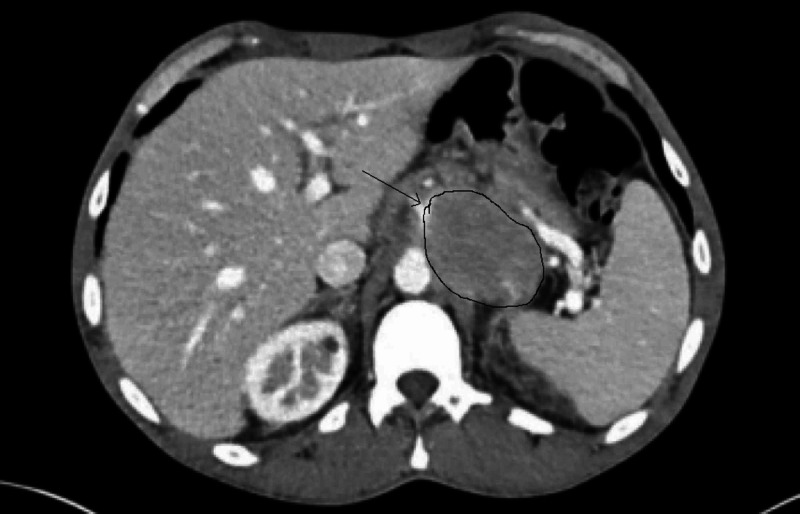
CT scan of the abdomen Contrast-enhanced CT abdomen demonstrating a mildly enhancing soft tissue mass in the lesser sac invading the splenic artery.
